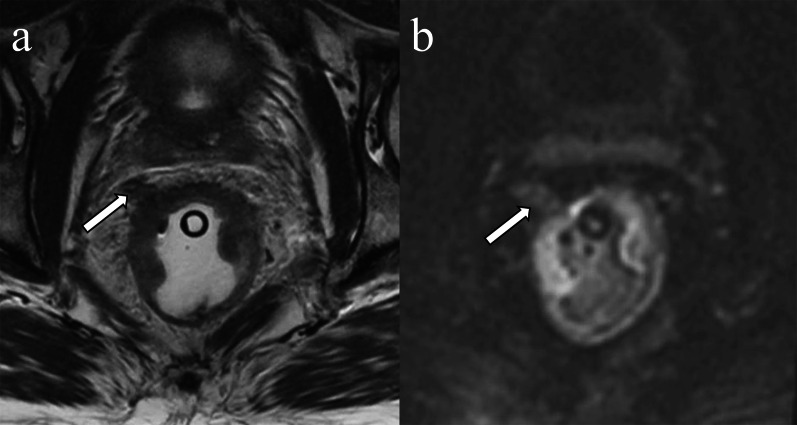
Extramural invasion on diffusion weighted imaging. A 41-year-old man with rectal adenocarcinoma. T2-weighted image demonstrates a distended perirectal vessel with a lack of flow void and central tumor signal intensity which is contiguous with the primary rectal tumor (a arrow). Diffusion-weighted image shows a high signal intensity cord-like structure contiguous with the primary rectal tumor (b arrow)
